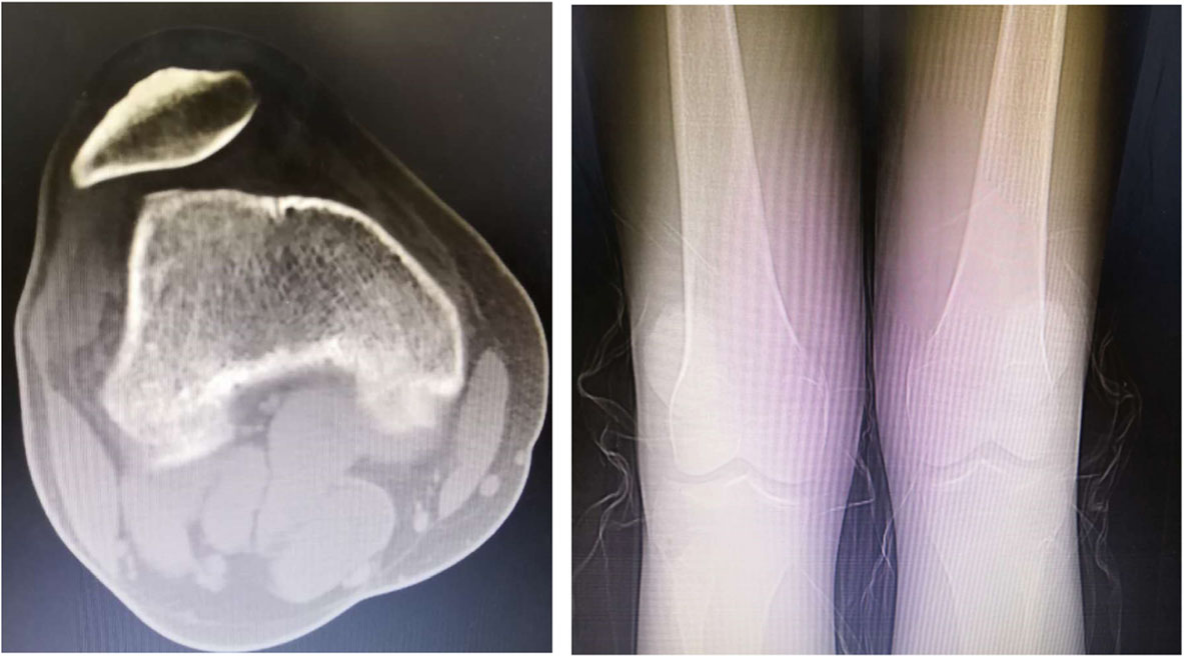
Preoperative CT and X-ray images show patellofemoral instability and femoral trochlear dysplasia
